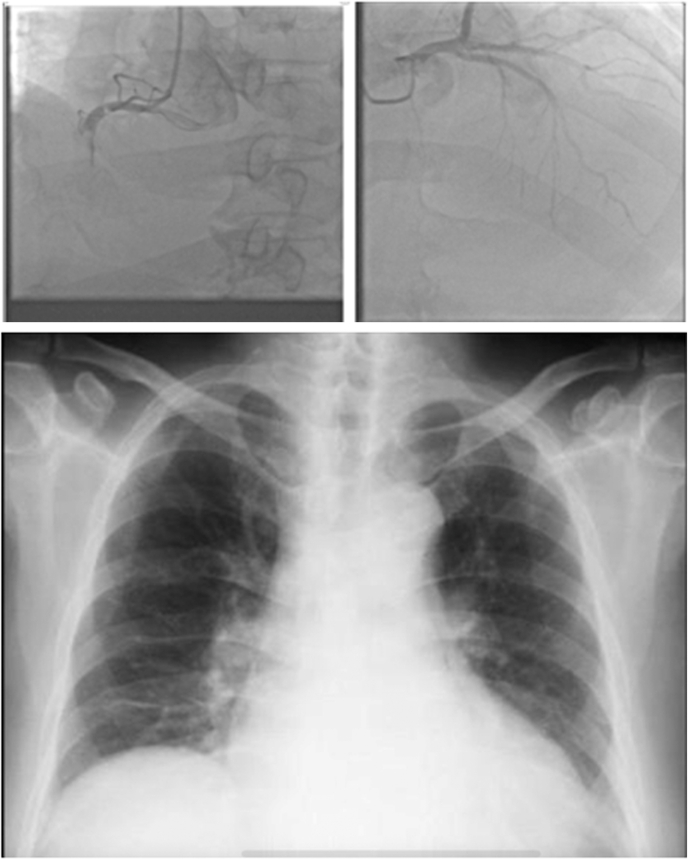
Preprocedural Coronary Angiography and Chest X-Ray
